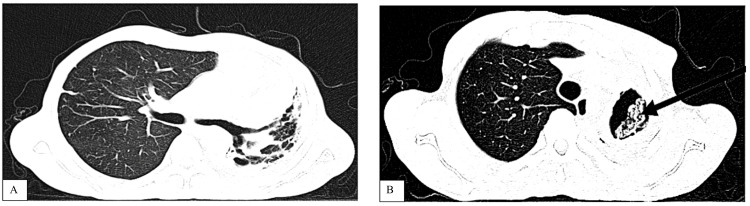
Chest CT-scan with the radiologist’s report. (A) Extensive left lung fibrotic changes with near total lung collapse and shift of the mediastinum to the left. Associated left lung bronchial wall thickening with dilatation. Left upper lobe cavitary lesion containing an irregular mass. Compensatory right lung hyperinflation with no obvious lesions. Trachea is slightly shifted to the left. No lymphadenopathy. Heart is not enlarged. No bone lesions. (B) Post-pulmonary tuberculosis changes showing bronchiectasis, left lung collapse, and left upper lobe cavitary lesion containing an irregular mass. Differentials for the irregular mass lesion within the cavity include necrotic lung tissue versus a mycetoma.
